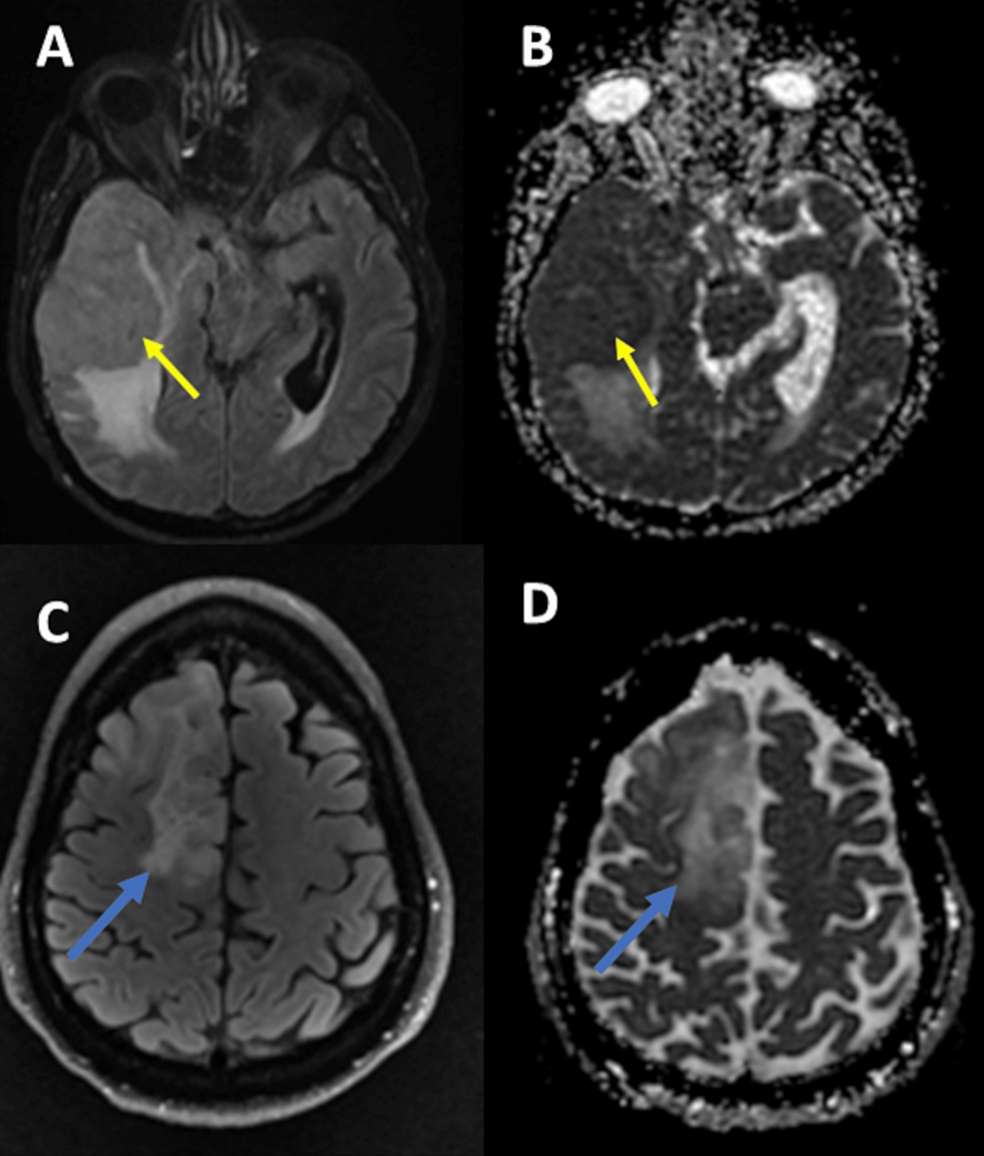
Role of DWI in differentiating between different brain tumors. (A, B) The images represent a right cerebral convexity atypical meningioma  (yellow arrow). (C, D) The images represent a right frontal lobe oligodendroglioma  (blue arrow). (A) FLAIR MRI shows a right cerebral convexity atypical meningioma with ventricular trapping. (B) ADC map from DWI data depicting reduced diffusivity. (C) FLAIR MRI shows a  right frontal lobe oligodendroglioma. (D) ADC map from DWI data shows increased diffusivity (shine-through artifact).
